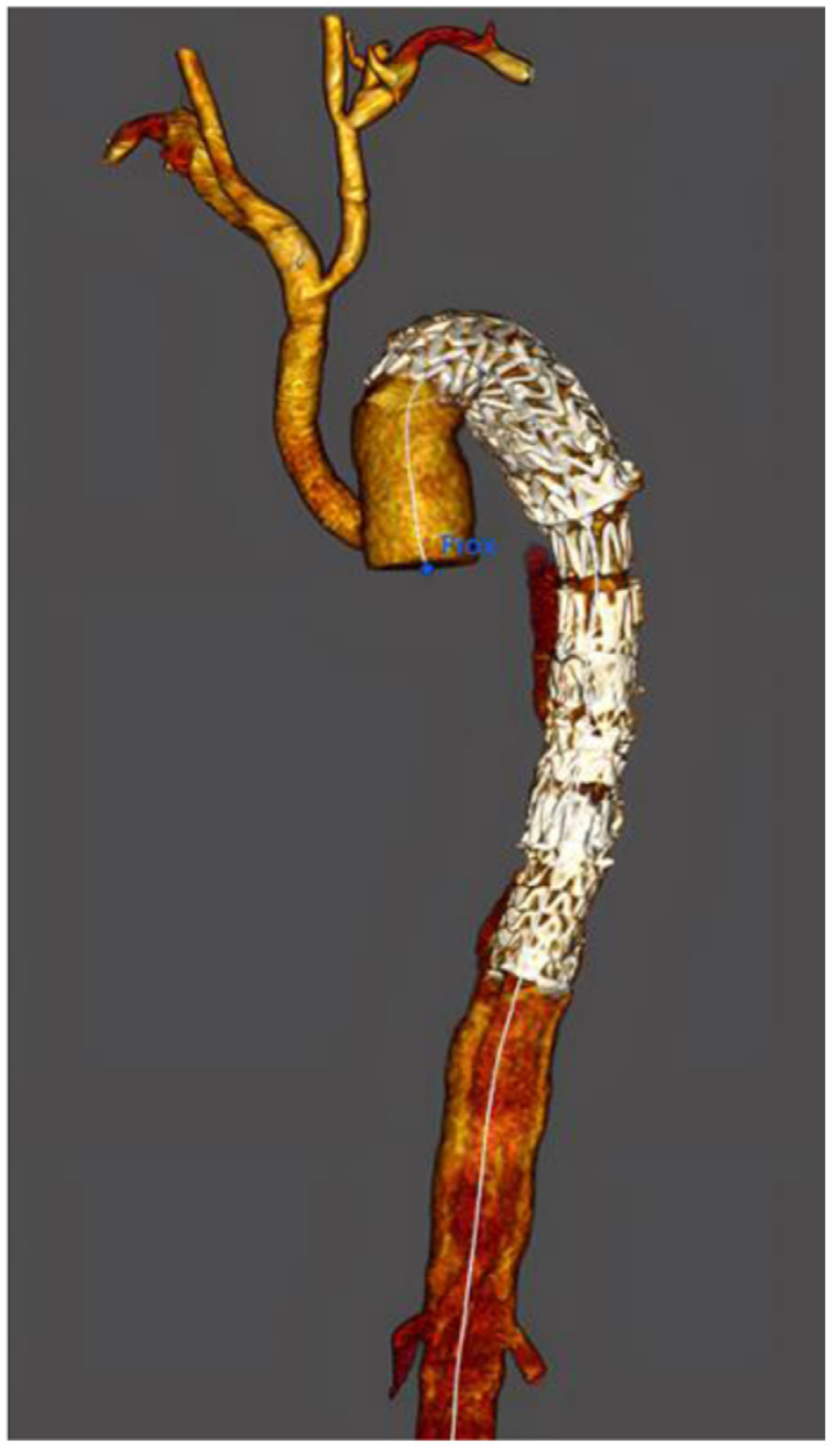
Three-dimensional computed tomography angiogram (CTA) at 6 months of follow-up demonstrating the aortic reconstruction showing the left subclavian to carotid transposition and ascending aortic replacement with reimplantation of the innominate artery by a bypass graft.
